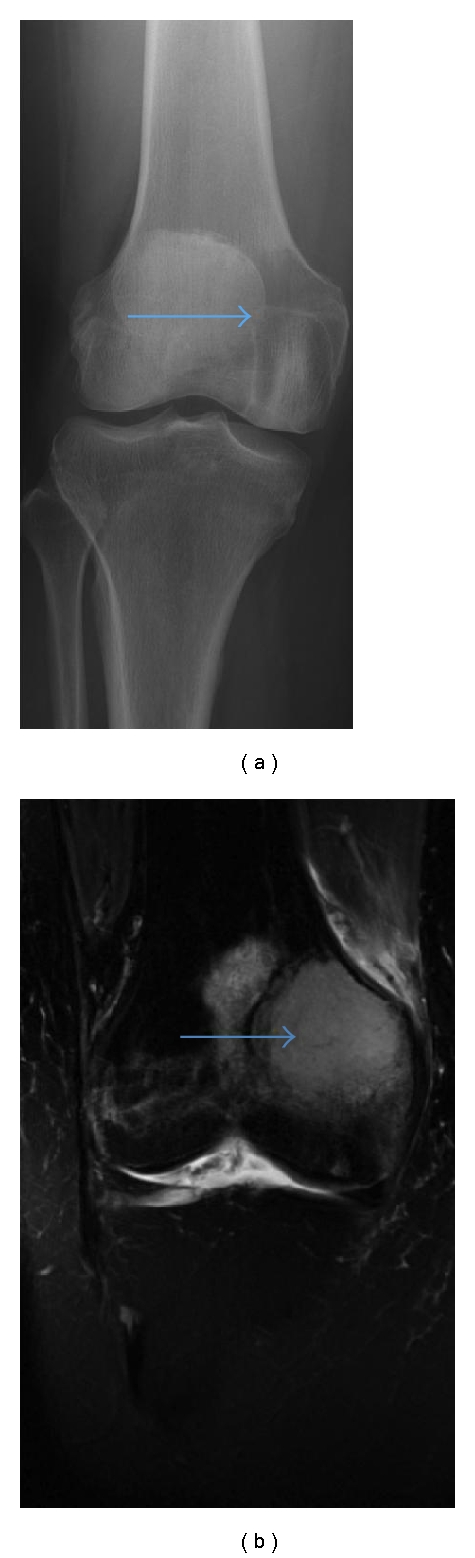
A-P radiograph right knee (a): 4 cm lucency medial femoral condyle (arrow), radiographically difficult to visualise. MRI coronal T2-weighted STIR sequence (b, same patient): high signal 4 cm plasmacytoma medial femoral condyle (arrow).
